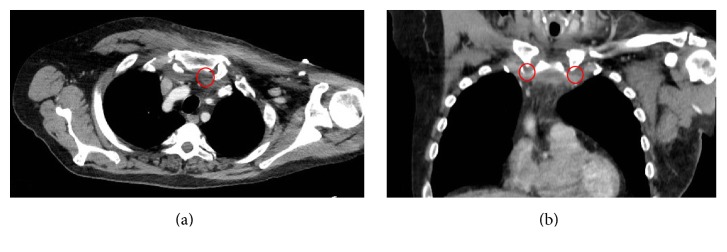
Chest CT scans. (a) Axial image; (b) coronal image showing evidence of bilateral sternocleidomastoid inflammation/infection. Thickened soft tissue was noted surrounding the SC joints and posterior to the sternum labelled by the red circle.
